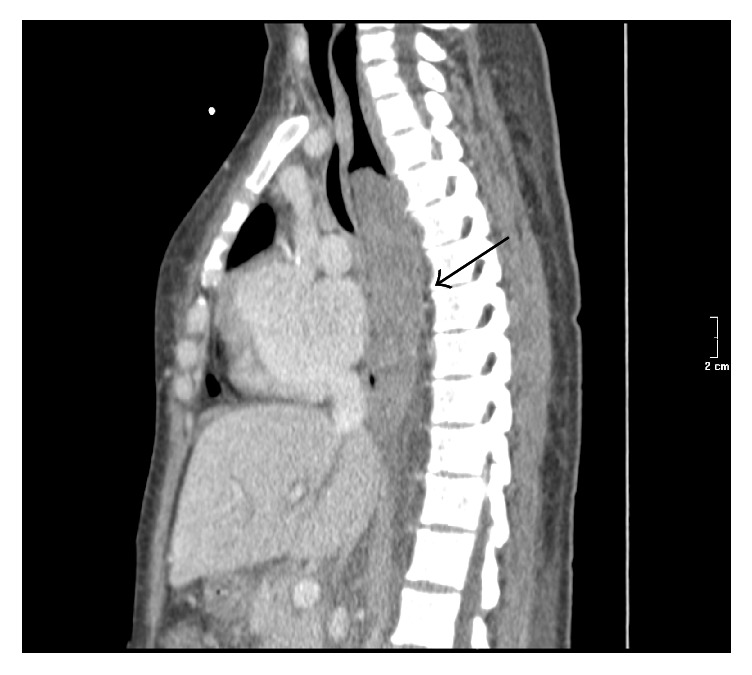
Second post-C-section day chest CT sagittal view of the esophageal hematoma (black arrow) infiltrating the posterior wall.
